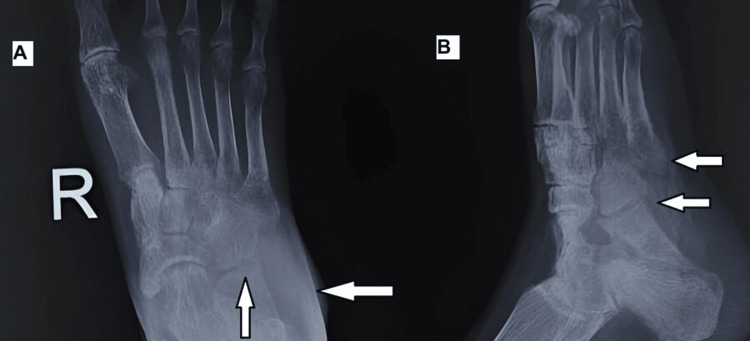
X-ray of the right foot in anteroposterior and oblique views. Image A (arrows) shows marginal erosion and soft tissue swelling. Image B (arrows) shows periarticular osteoporosis and joint space narrowing with subluxation of the cuboid.
